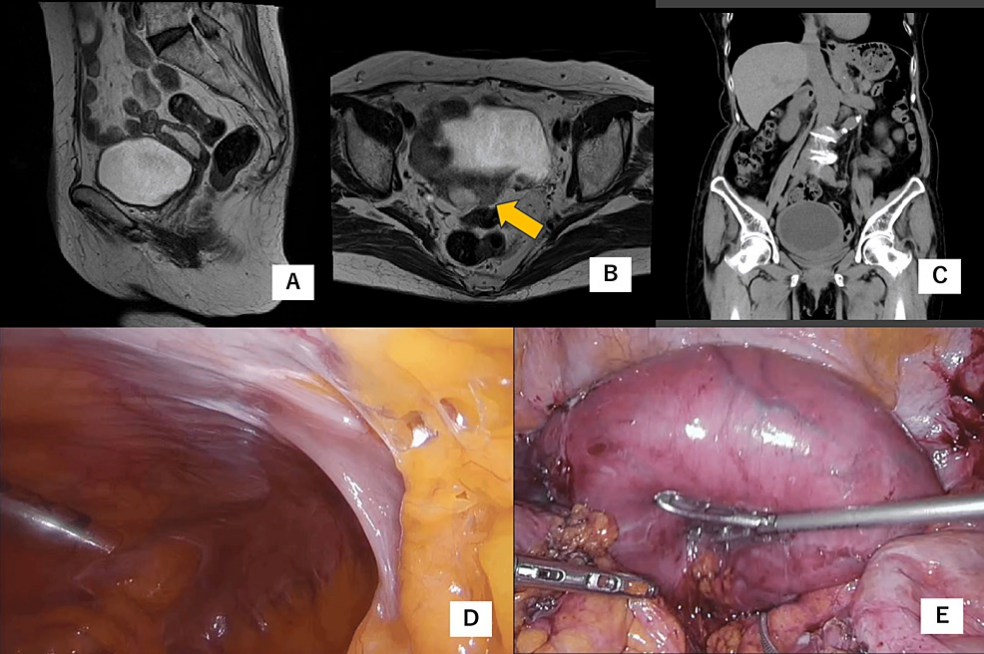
Preoperative imaging studies and laparoscopic surgery (A and B) Magnetic resonance image (T2 image) acquired after drainage. (A) A small amount of fluid was retained in the uterine cavity. (B) The arrow shows a lesion on the left wall of the uterine body with a pale high-signal. This lesion is high-signal on diffuse-weighted imaging and its apparent diffusion coefficient is low (not shown). (C) Computed tomography performed on the day before surgery. The uterus was enlarged with hematometra. (D and E) Laparoscopic surgery. (D) The small intestine was adherent to the abdominal wound. (E) Enlarged uterus. Pelvic peritoneal dissemination was not observed.
